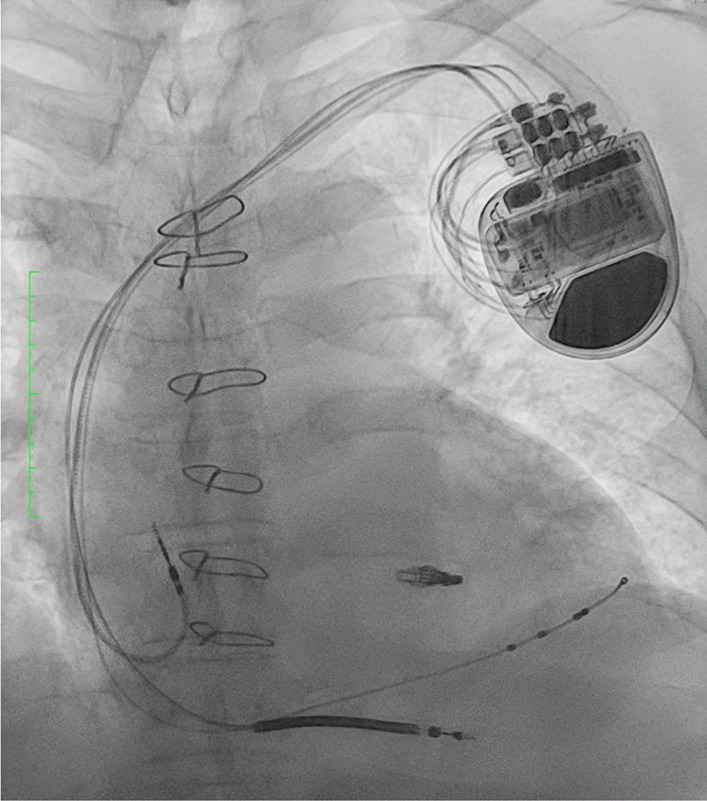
Postoperative chest radiograph after cardiac resynchronization therapy-defibrillator placement
